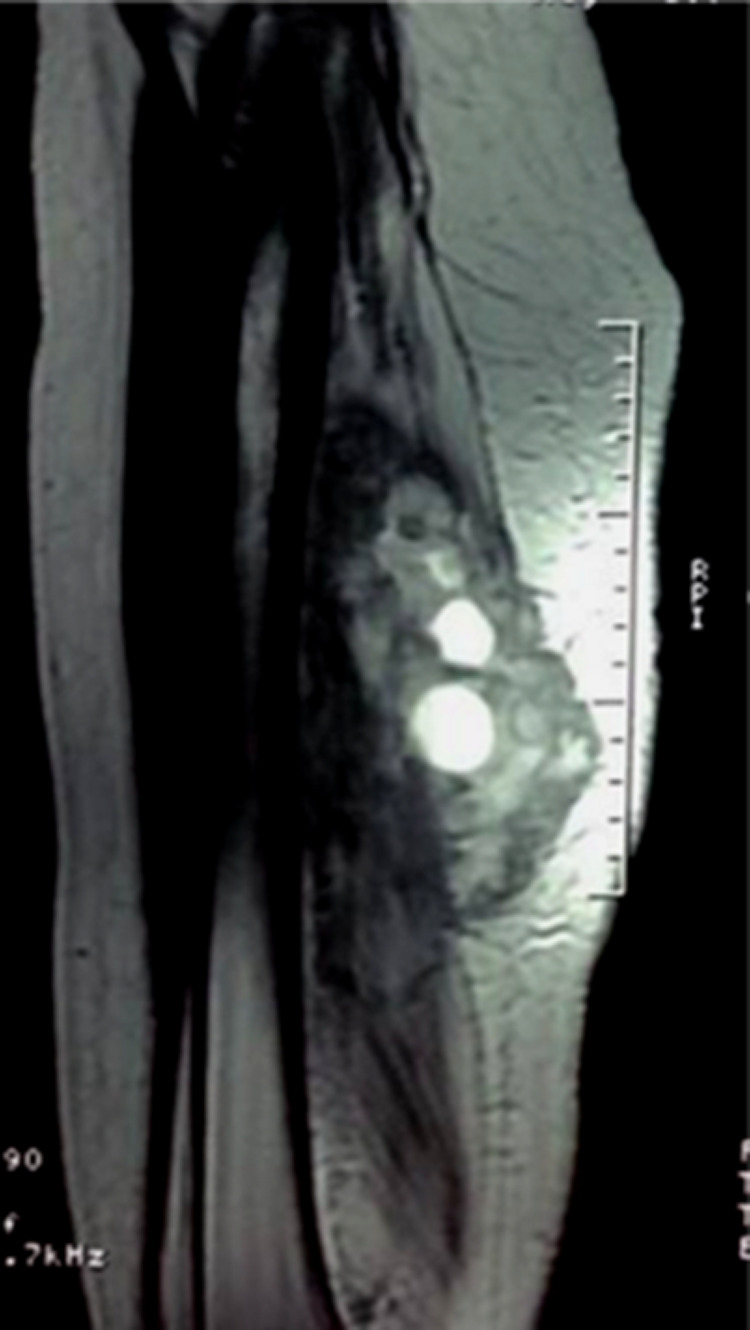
MRI of the left thigh: Sagittal TSE T2-weighted image shows the multiloculated cystic lesion with multiple daughter cysts in the middle one-third of the posterior compartment of thigh.
